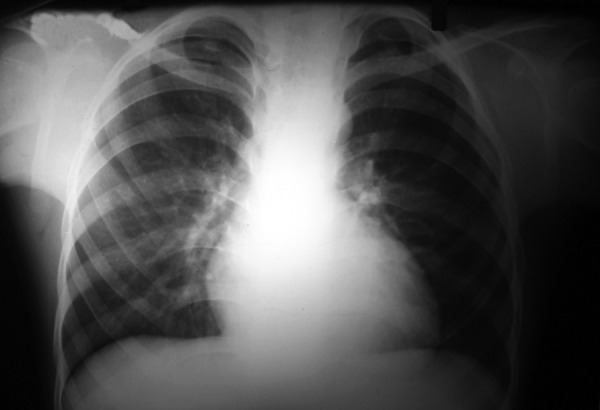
CT of skull with calcification
